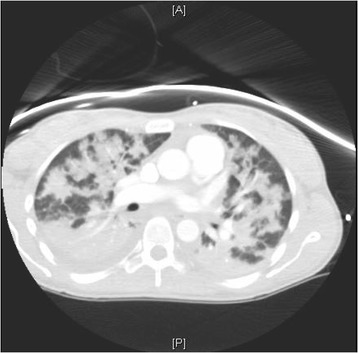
Chest CT with IV contrast obtained at the onset of respiratory insufficiency, showing new diffuse bilateral heterogeneous and consolidative opacities with small right greater than left pleural effusions, consistent with ARDS
